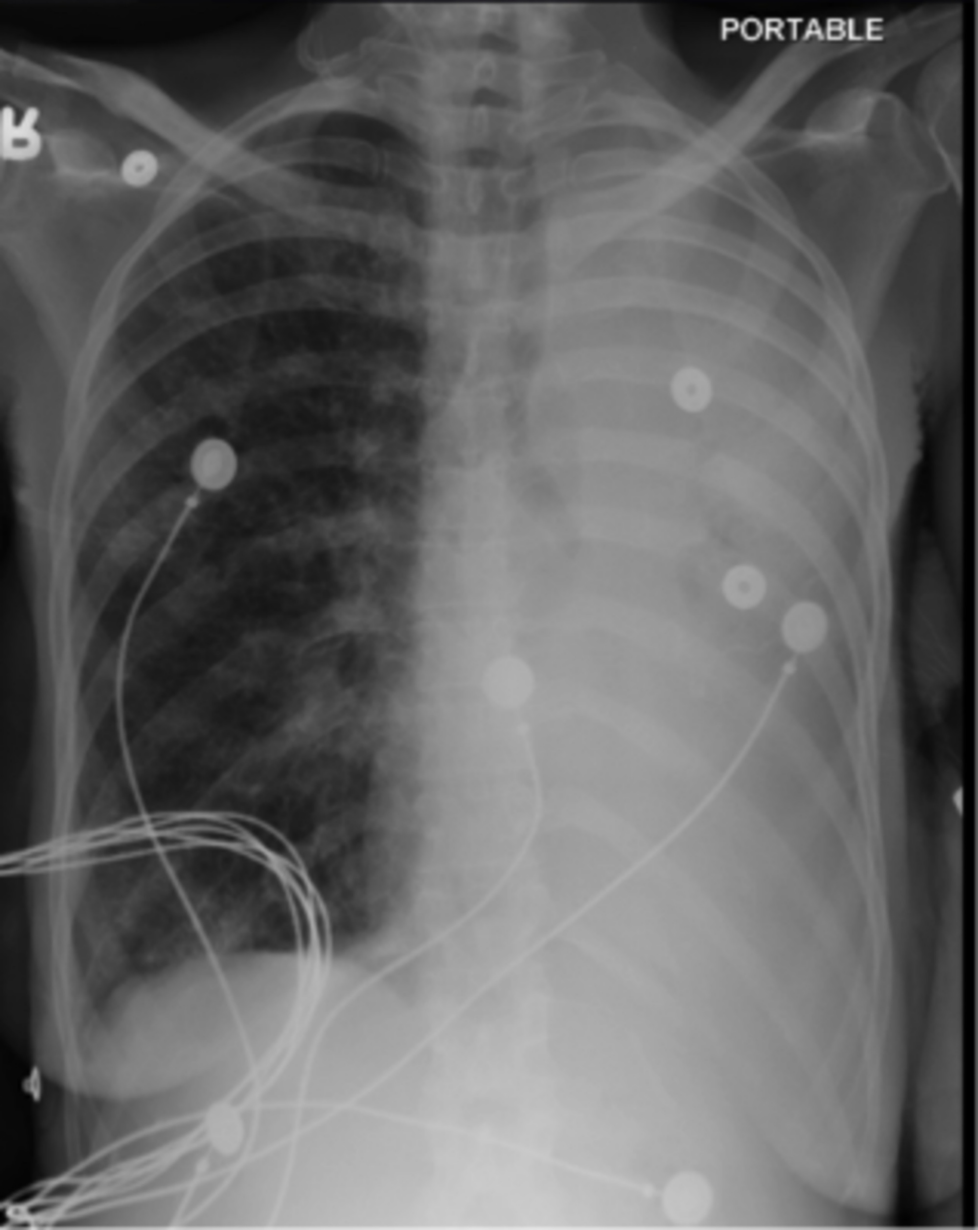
Chest X-ray showing opacification of the left hemithorax
